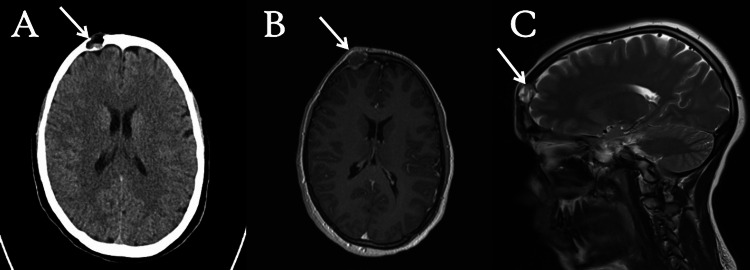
Preoperative imaging including MRI and CT scan. (A) CT scan without contrast shows an expansive soft tissue mass with bevelled edges, measuring approximately 1.5 x 1.8cm in the right frontal bone. Notably, the mass reaches the surface of dura without infiltrating the brain parenchyma (white arrow). (B) T1-weighted MRI axial view reveals a hypo-intense lesion on the right frontal skull lesion (white arrow). (C) T2-weighted MRI sagittal view reveals tumor impingement of the dura (white arrow). CT: computed tomography; MRI: magnetic resonance imaging
